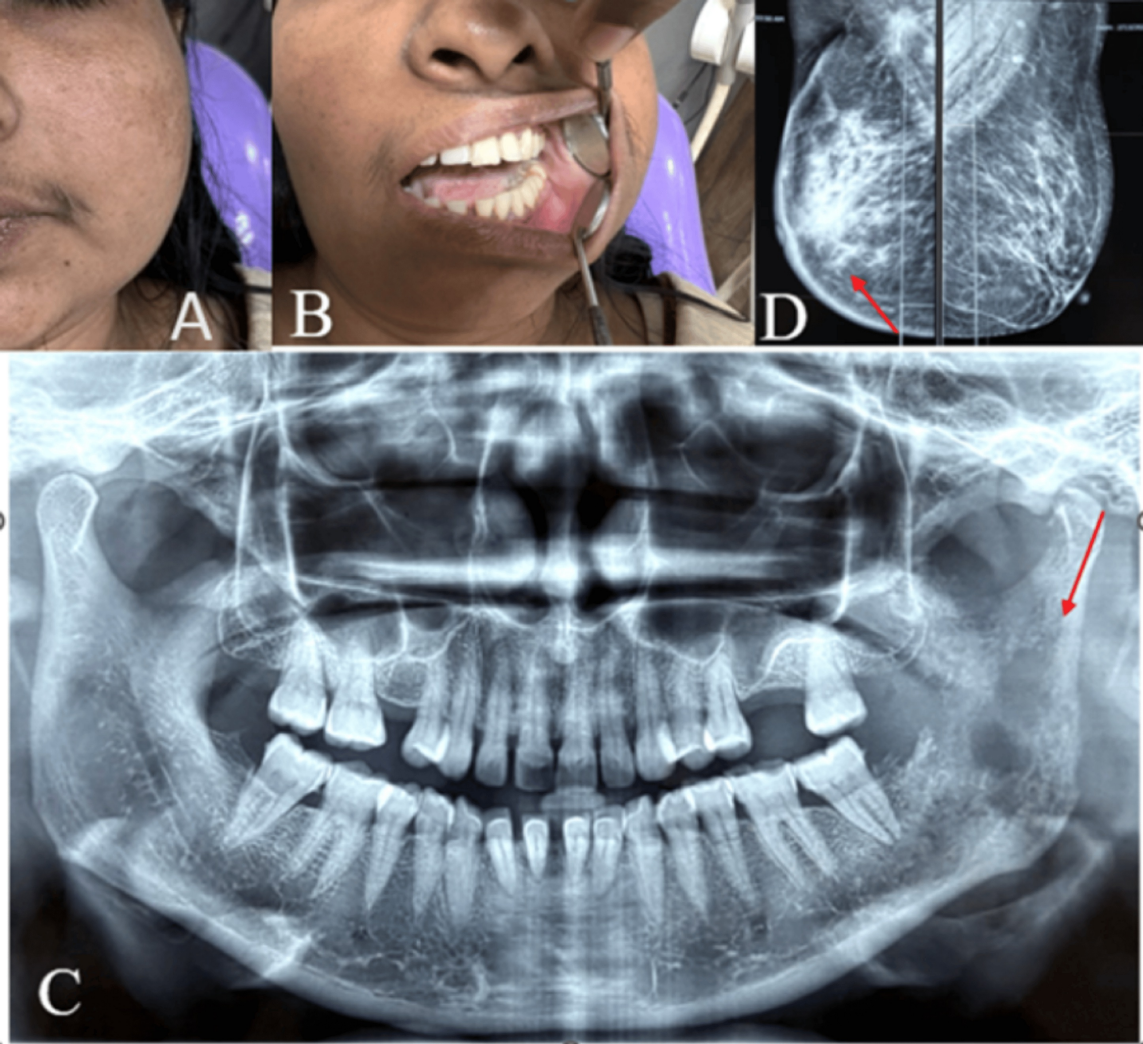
Mandibular metastasis of breast carcinoma. A: Extraoral picture shows a diffuse swelling on the left lower jaw. B: Restricted mouth opening. C: Panoramic radiograph shows multiple ill-defined lytic areas. D: Mammogram shows an irregular high-density lesion in the retroareolar region of the right breast
